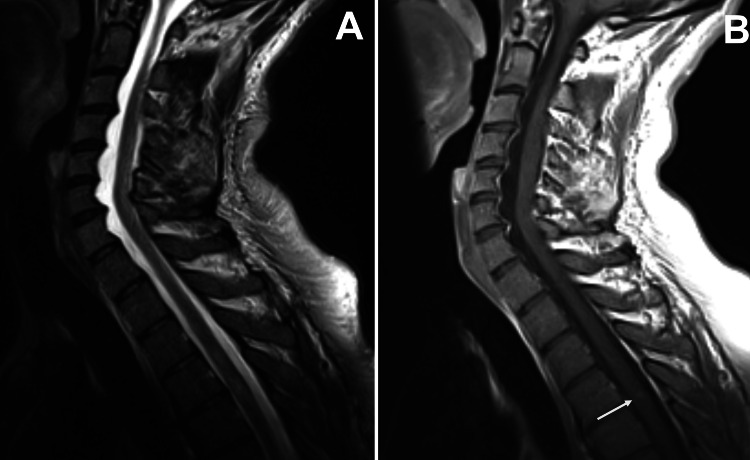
Spinal cord magnetic resonance imaging. (A) On a sagittal view of a T2-weighted turbo spin echo sequence, several hyperintense lesions involving the spinal cord can be observed; (B) one of them mildly enhances after administration of gadolinium on a sagittal view of a T1-weighted turbo spin echo sequence (arrow). Their location and morphology are atypical for areas of signal intensity (FASI) and could be related to a demyelinating process.
